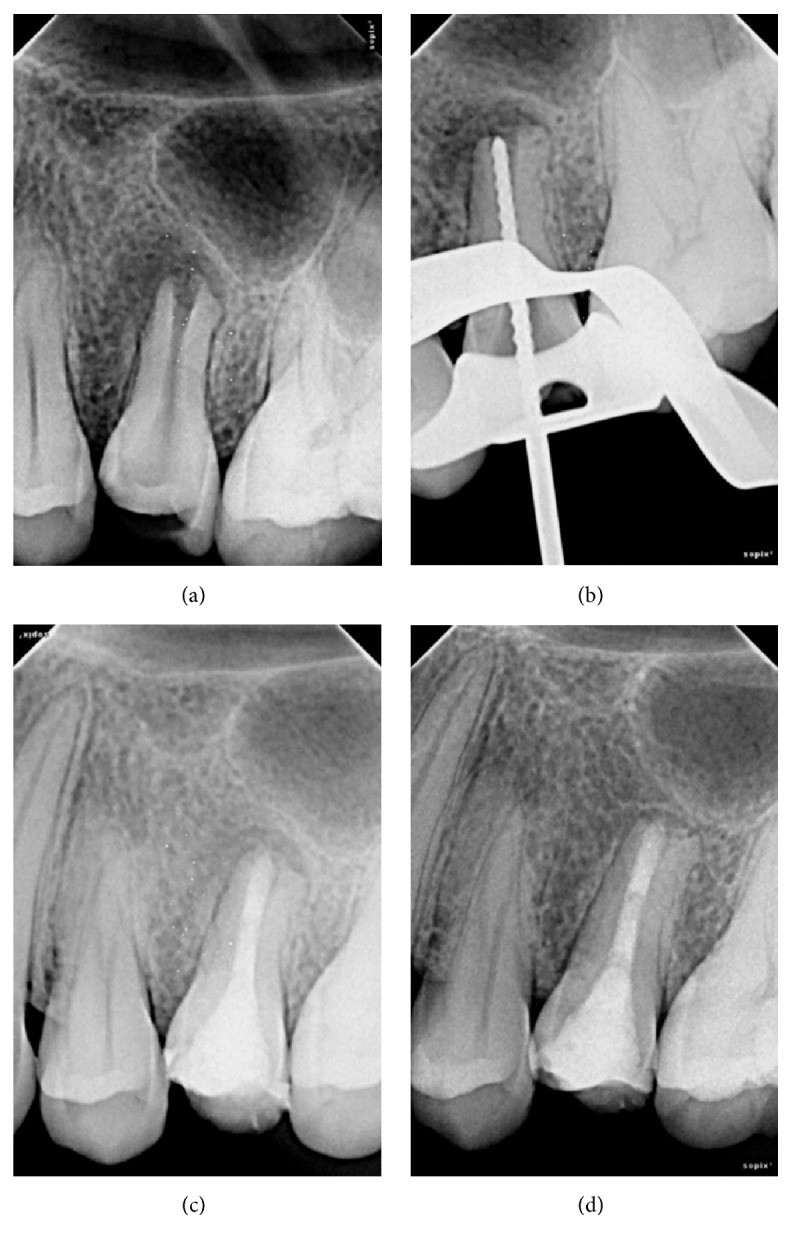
(a) Preoperative radiograph (Case  2). (b) Working length radiograph (Case  2). (c) MTA obturation (Case  2). (d) One-year follow-up radiograph (Case  2).
